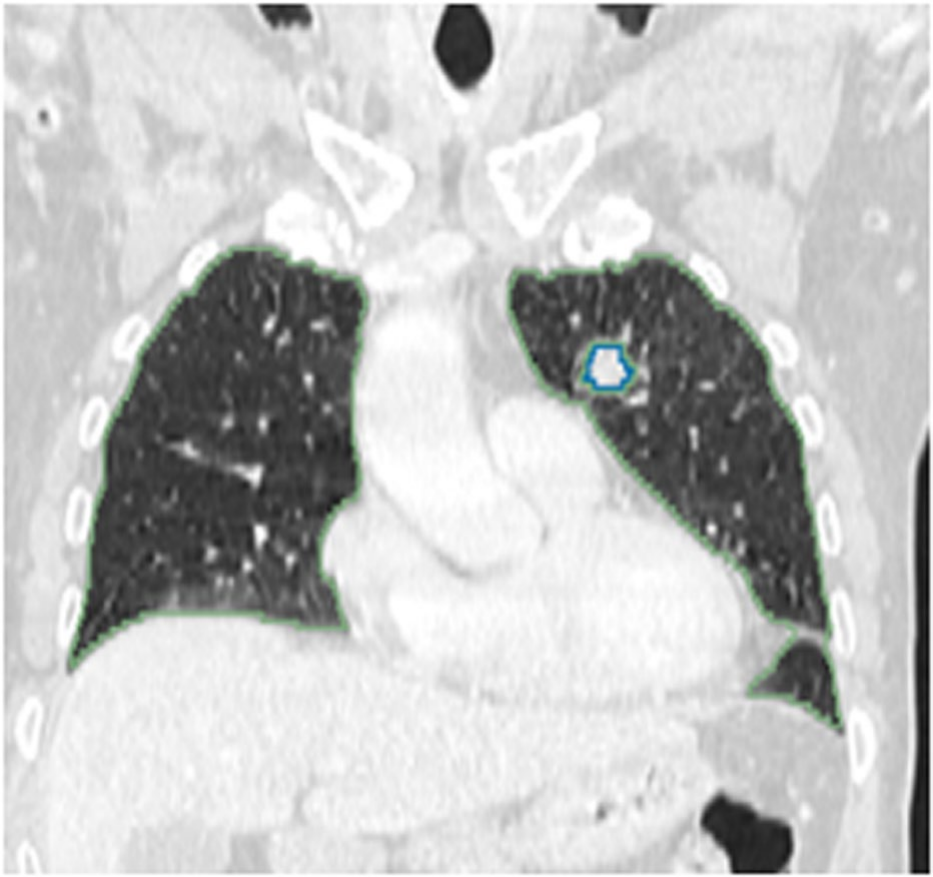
Automatic segmentation of Lung (green) and nodule (blue) from CT scan of patient TCGA-34-5239.
